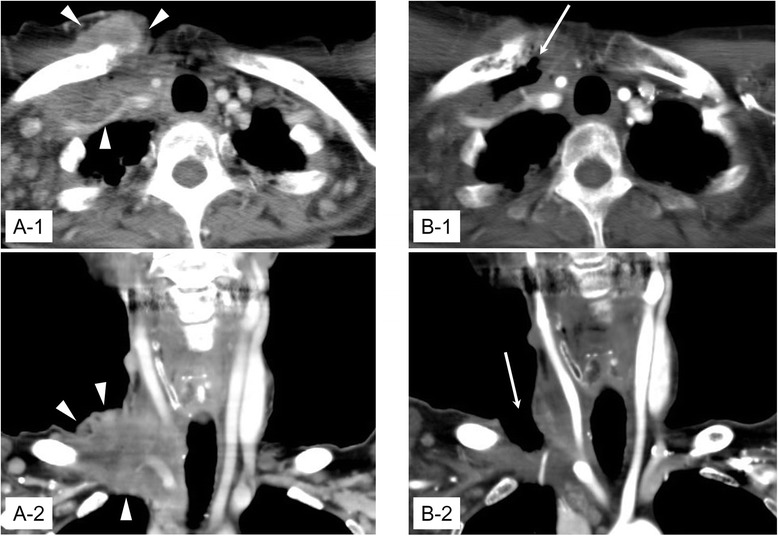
A-1, A-2 Enhanced CT findings before starting lenvatinib. Tumor is invaded into subcutaneous tissue in the right subclavicular lesion (*arrowhead*). B-1, B-2 Enhanced CT findings just before surgery (day 32). A large ulcer occurred in right subclavicular lesion (*arrow*)
